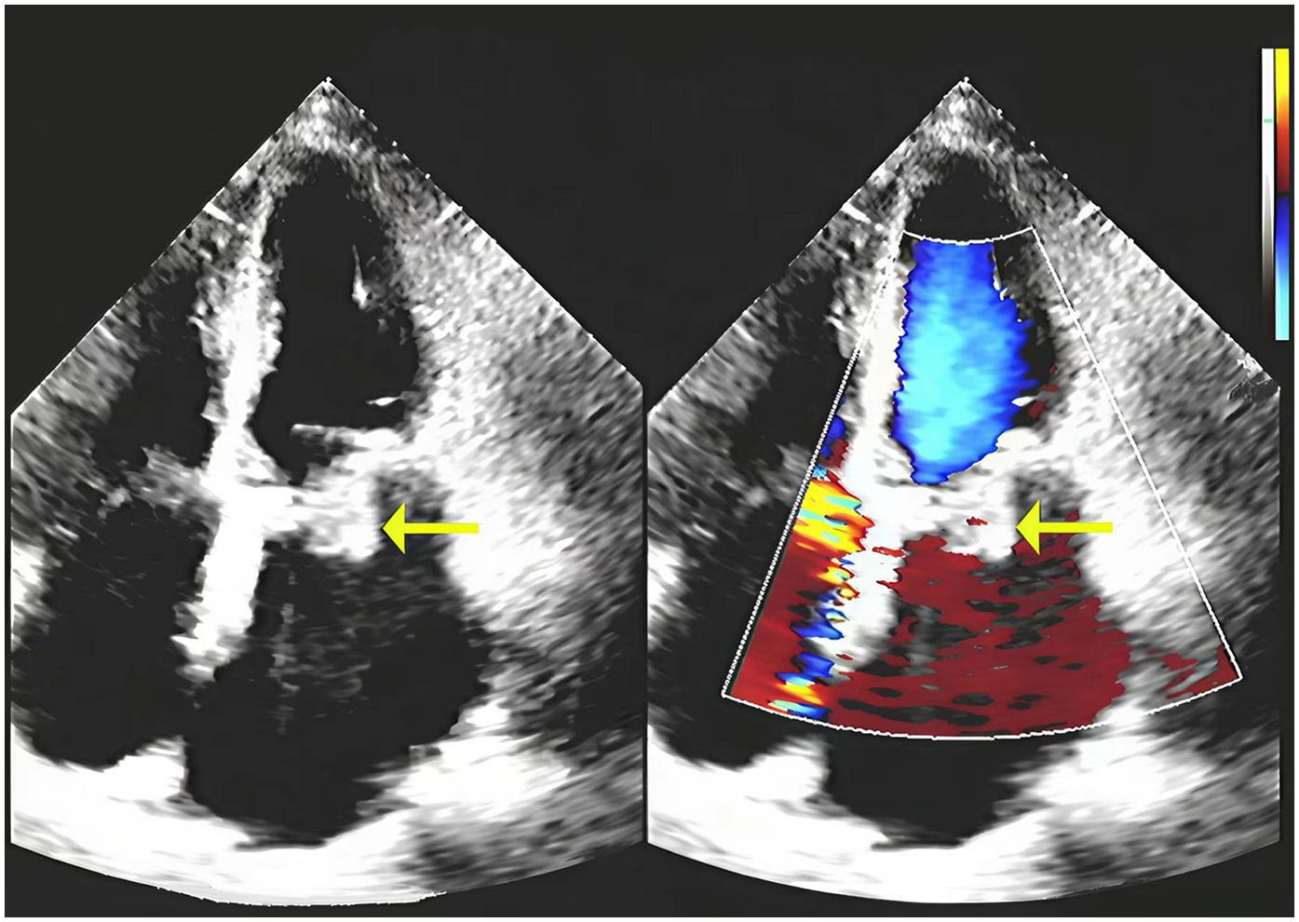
Transthoracic echocardiography (left panel) and color Doppler image (right panel) demonstrate a vegetation on the mitral valve (yellow arrow).
